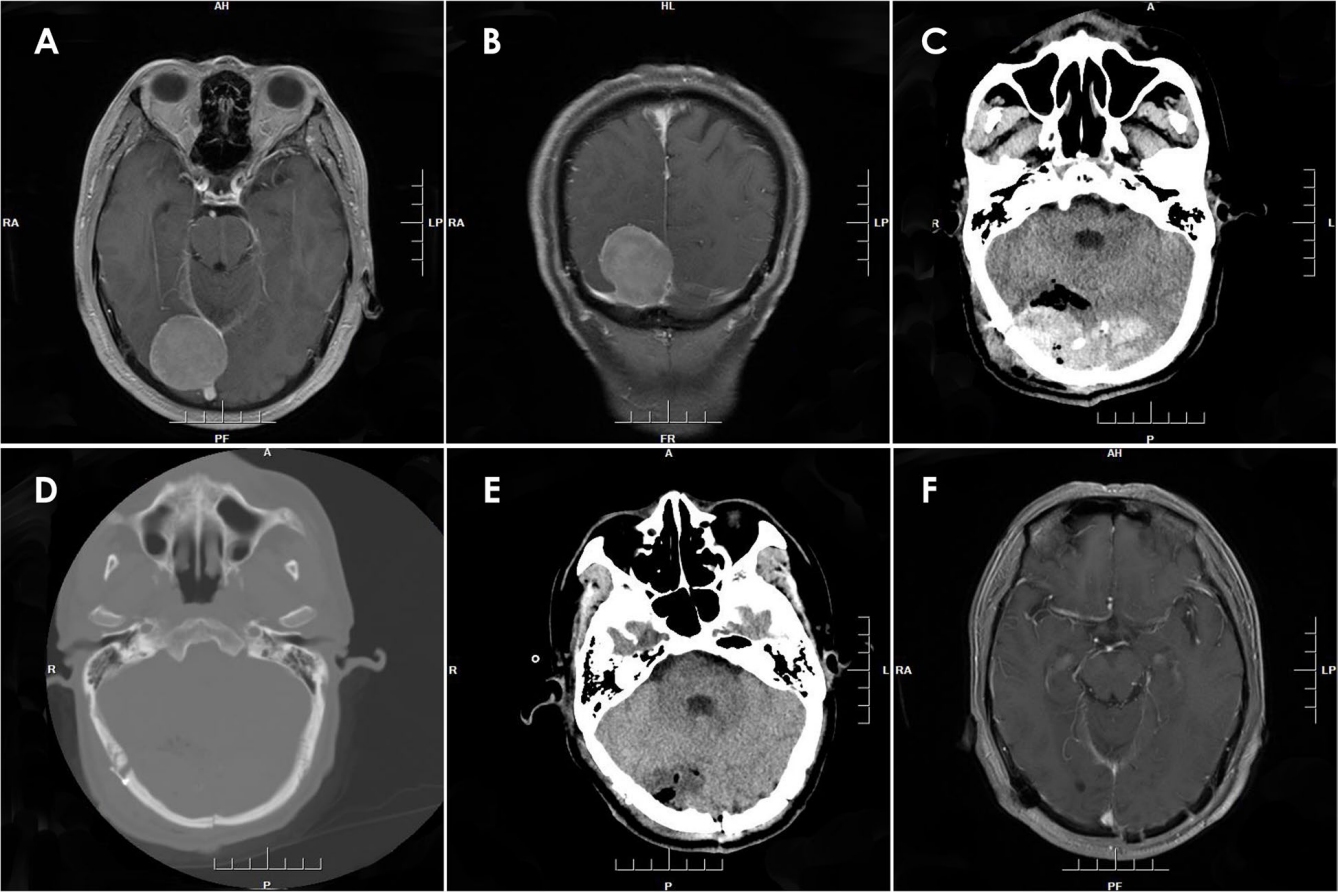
MRI with contrast enhancement revealing a right peritorcular meningioma (A and B). A type 2 PEDH on the bilateral occipital lobe (C). The causes of PEDH were attributed to the failure of dura suspension and bleeding in the sagittal sinus, according to the operative note. The CT bone window showed that the bone flap reached the midline, possibly leading to incomplete suspension due to the sagittal sinus (D). The EDH was evacuated (E). MRI with contrast enhancement obtained 3 months after surgery (F)
